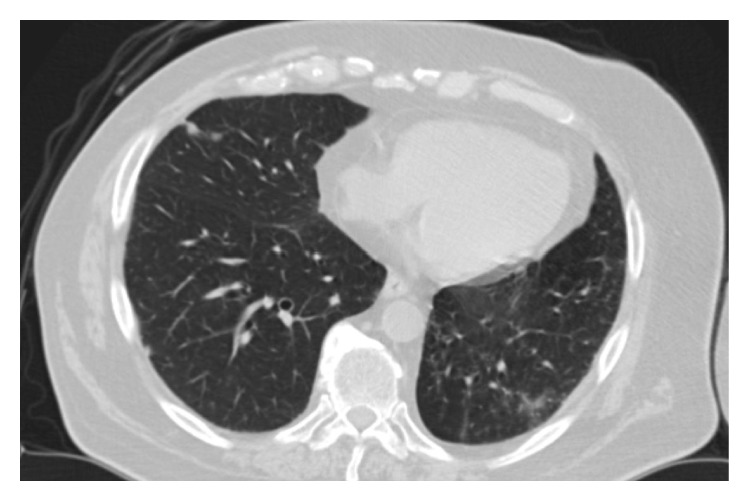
Computed tomography scan of the thorax showing right-sided pulmonary metastases approximately 3 weeks after radiotherapy to other targets. Marked reduction in size.
